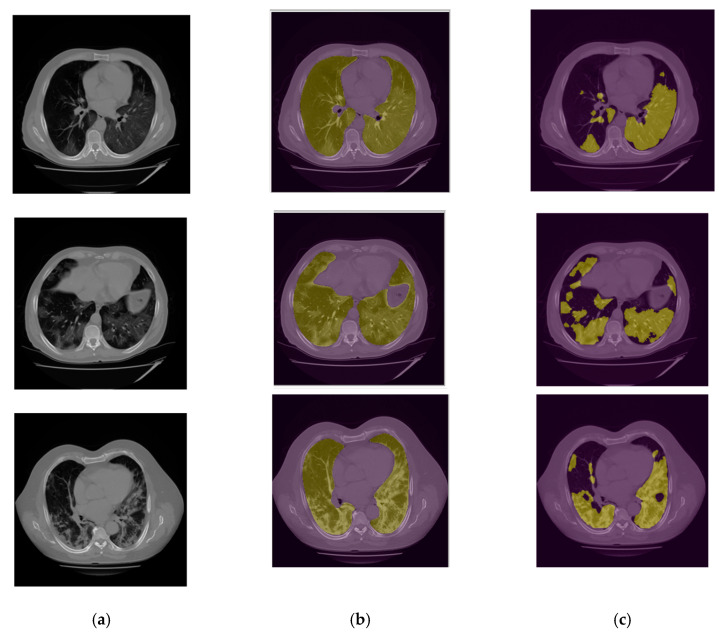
(a) Raw CT image; (b) Lung mask; (c) Infection Segmentation.
